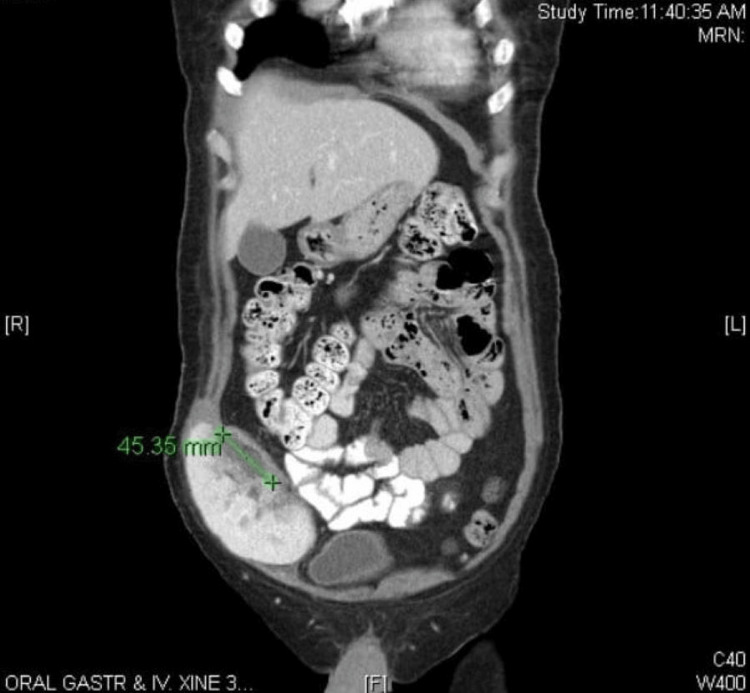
CT angiography of the pseudoaneurysm and anastomosis of the transplanted renal artery.
